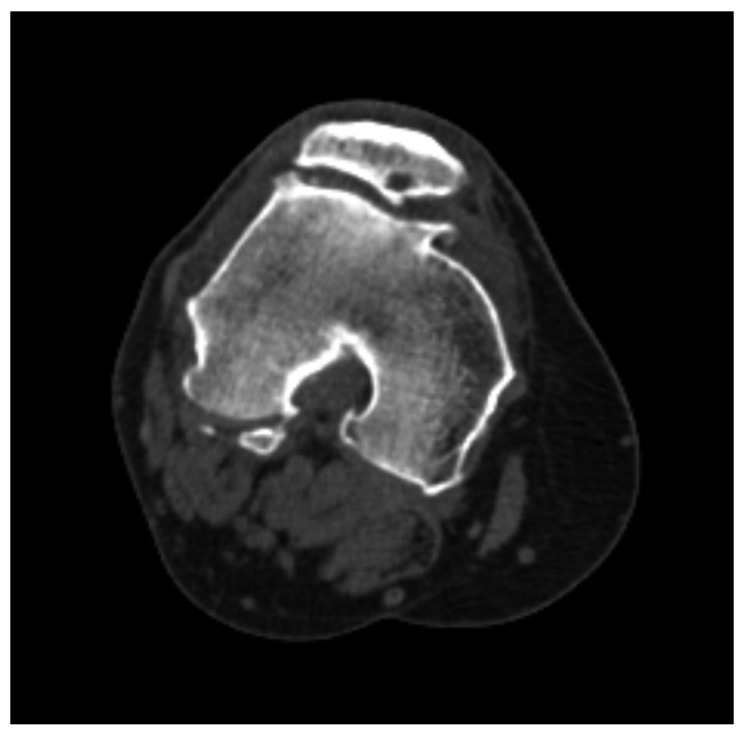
Axial CT image of the knee demonstrating advanced secondary osteoarthritic changes in the patellofemoral joint.
